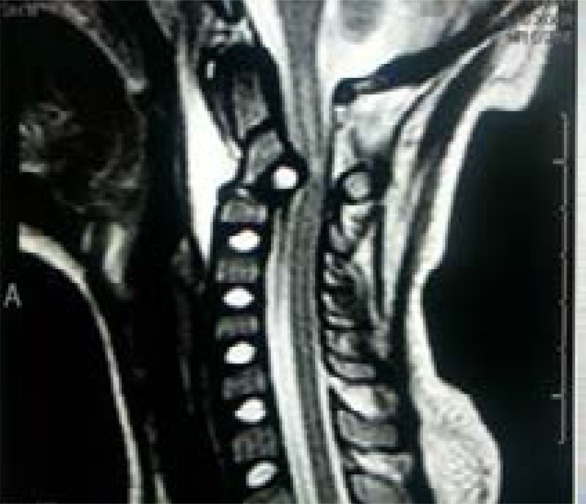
Sagittal cervical T2-weighted MRI showing abnormal high signal intensity extending from C1-C2/C3 disc space and increased prevertebral soft tissue swelling/fluid content.
